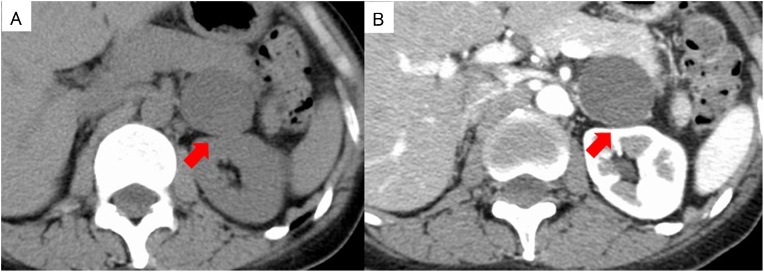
Computed tomography (CT) revealing a homogenous unilocular cystic mass between the pancreatic tail and the left adrenal grand, measuring 39 mm in the largest diameter (red arrow) (A). The wall of the cystic lesion is slightly enhanced and there are no solid components or calcification (red arrow) (B).
